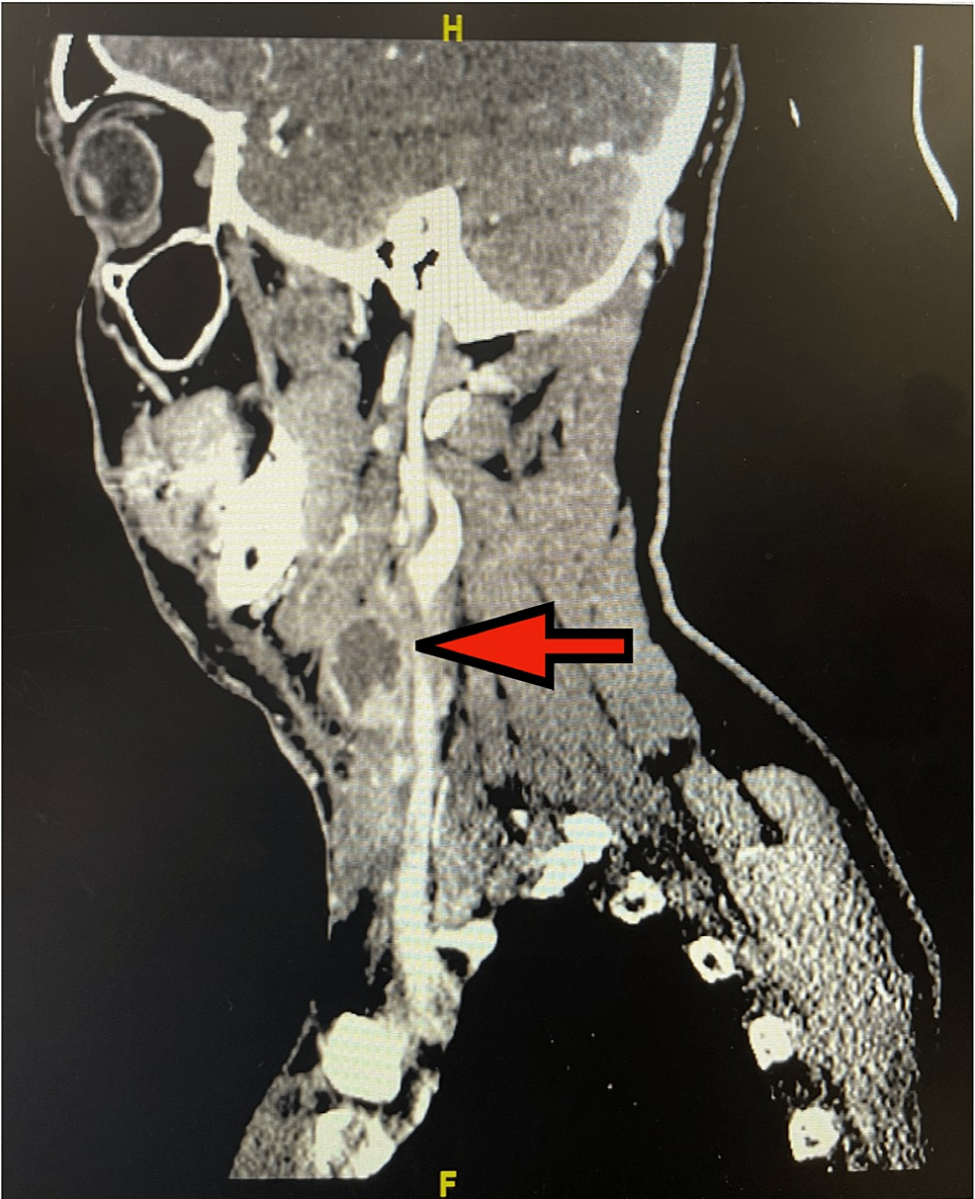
Sagittal view CT scan. Red arrow shows left neck mass, likely lymphatic in origin. CT: computed tomography
